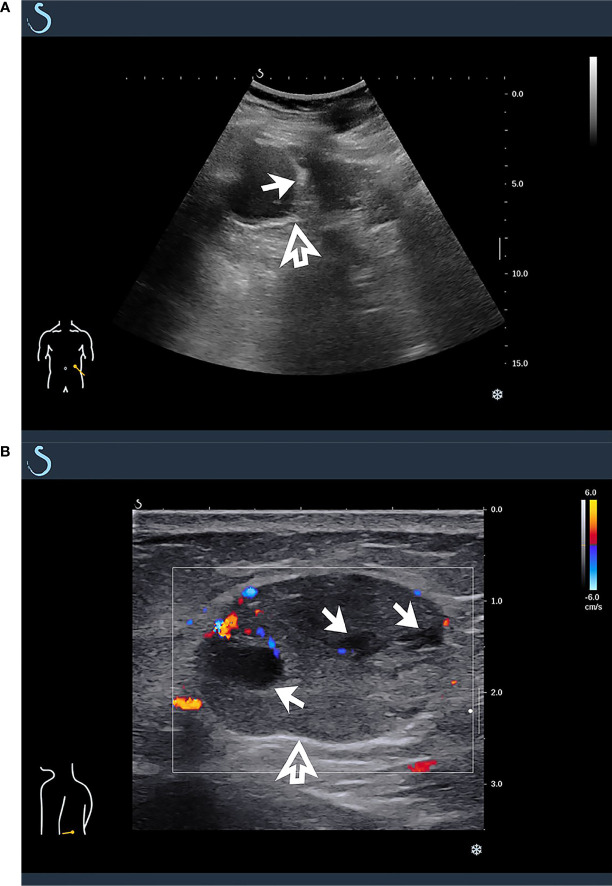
Ultrasonographic findings of masses in retroperitoneum and abdominal wall. (A) A multicystic mass was found in retroperitoneum of left renal region by grayscale using low-frequency transducer (hollow arrow), which featured multiple septa inside the cystic component (solid arrow). (B) By high-frequency probe, a hypoechoic mass was detected in abdominal wall near the left waist, and there were punctiform and linear blood flow signals in color Doppler (hollow arrow), which featured patchy anechoic areas inside the mass (solid arrow).
